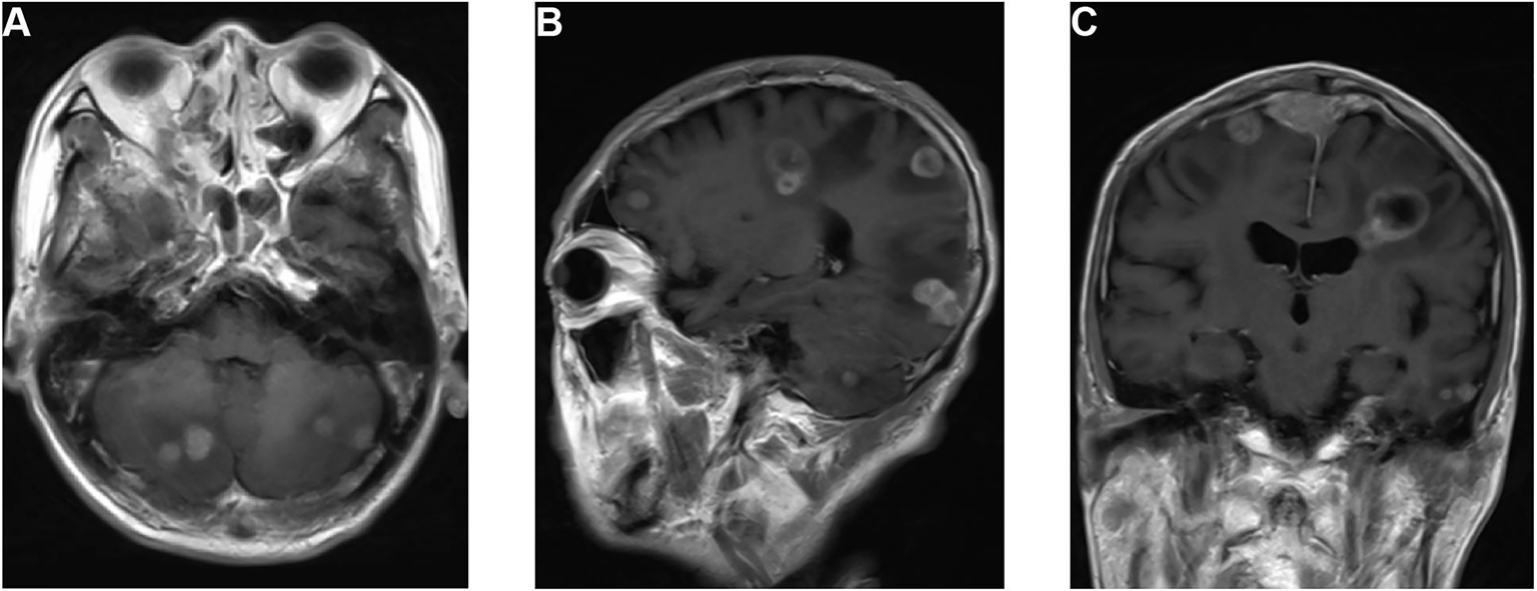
MRI of the dural and multiple brain metastases. (A) T1-weighted axial image shows cerebellum lesions. (B) T1-weighted sagittal image shows lesions in the cerebellum, occipital lobe, parietal lobe, and frontal lobe. (C) T1-weighted coronal image shows dural metastasis and lesions in the parietal and temporal lobes.
